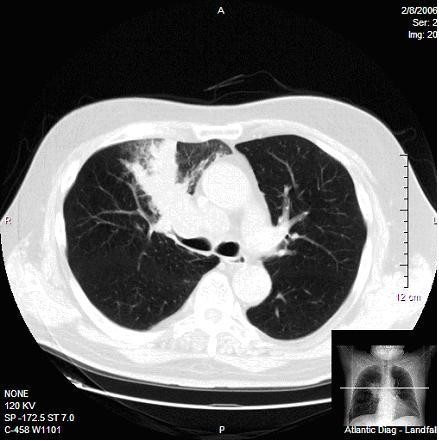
CT of the chest reveals a large mass in the right upper lobe of the lung with associated mediastinal and hilar adenopathy.
